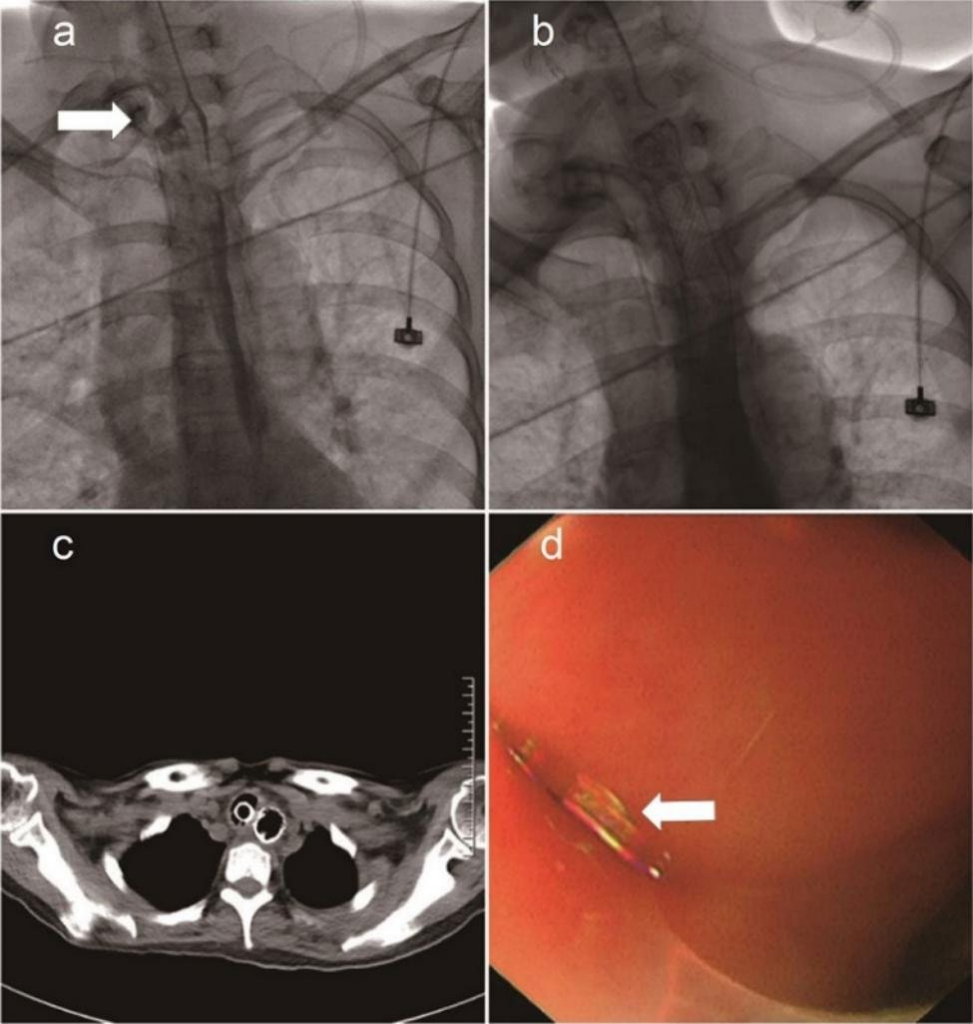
Oesophageal stent placement to treat ETF. The oesophagogram via a catheter demonstrates the communication (arrow) of the oesophagus and trachea (a). After stent placement, the fistula was occluded, and no contrast agent flowed into the trachea when the oesophagogram was performed via the catheter. CT also demonstrates successful occlusion (c). After the oesophageal stent was removed, a fistula (arrow) was observed by oesophagogastroscopy
